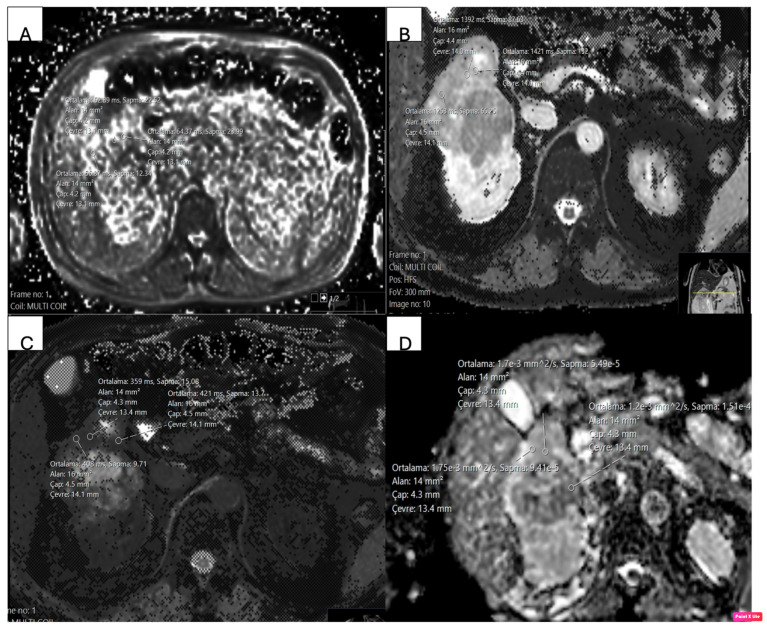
Quantitative MRI measurements obtained from renal tumor tissue. (A) T2* mDixon Quant image, (B) native T1 mapping, (C) post-contrast T1 mapping, and (D) ADC map. Regions of interest (ROIs) were placed within the solid components of the tumor, and mean values were calculated. R2* values were derived as the reciprocal of T2*.
